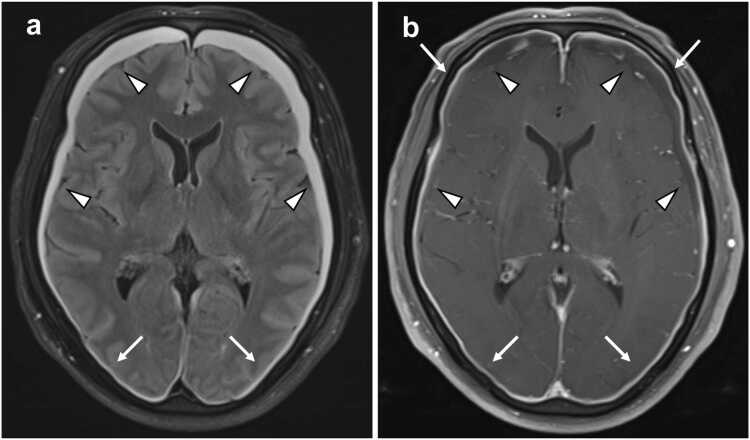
68-year-old male with dural/subdural lesions (the same patient shown inFig. 2). a Non-contrast FLAIR imaging: Diffuse pachymeningeal hyperintensity (arrows) and subdural effusion/hematoma (arrowheads) are observed, although the two cannot be clearly distinguished. b Post-contrast T1-weighted imaging: The dura mater demonstrates diffuse enhancement and thickening, indicative of diffuse pachymeningeal enhancement (arrows). Subdural fluid without contrast enhancement is present, corresponding to subdural effusion/hematoma as identified on non-contrast FLAIR imaging (arrowheads). Abbreviations: FLAIR *fluid attenuated inversion recovery*.
